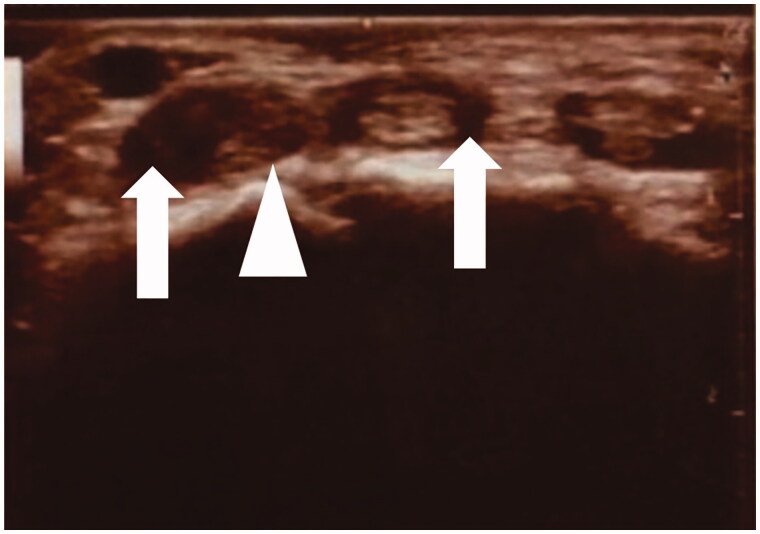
Preoperative diagnostic ultrasound imaging. A low echoic region (arrows) suggesting liquid was noted around the extensor digitorum communis (EDC) tendon and the 4th EDC tendon was not clearly visualized (arrow head).
